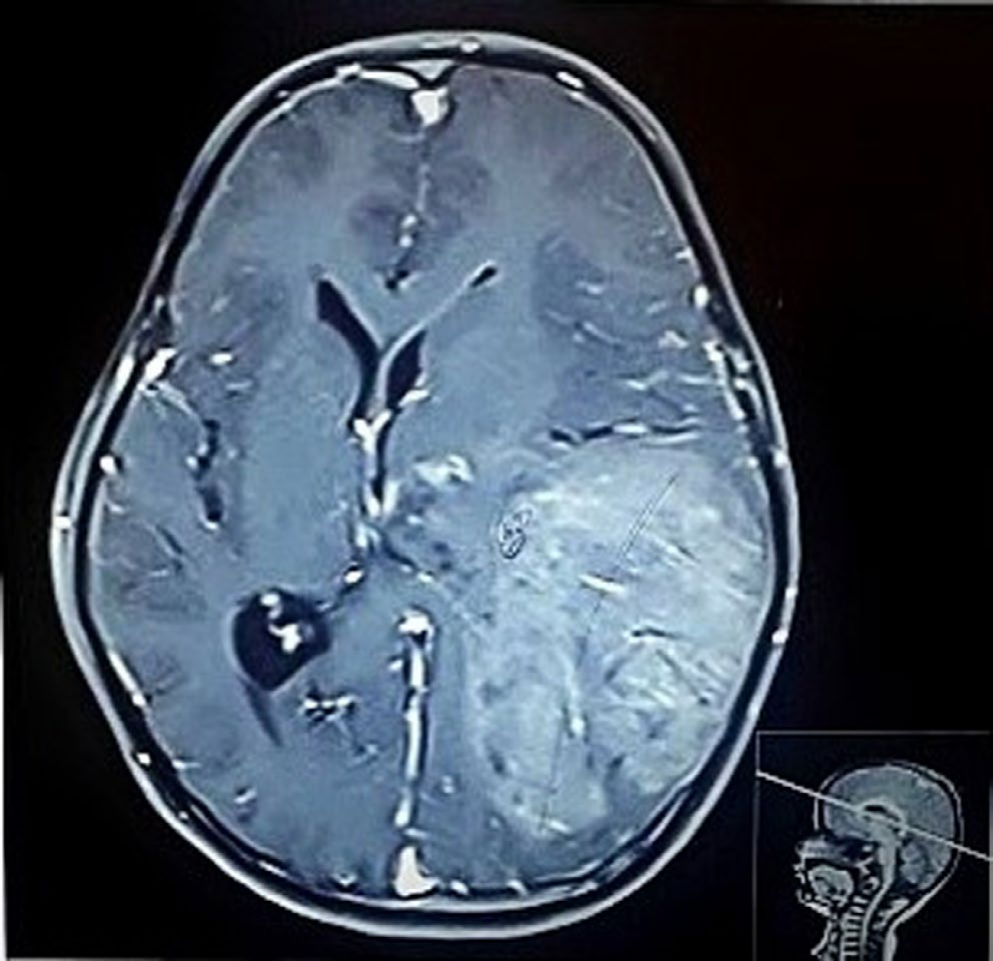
Contrast MRI brain showing a large heterogeneous enhancing lesion in left parieto‐occipital region reaching up to ventricle
